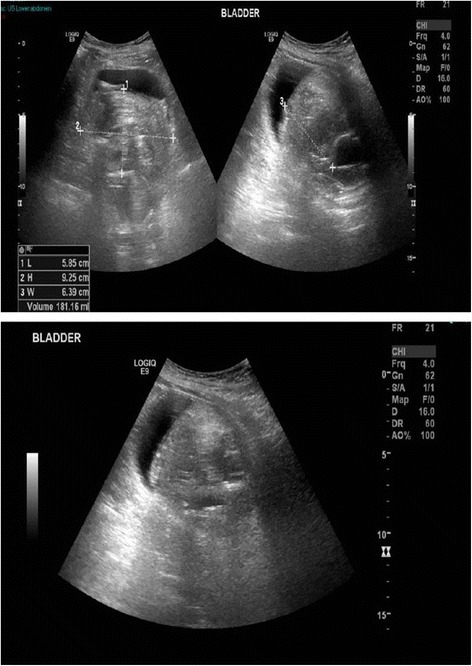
Ultrasound scan of urinary bladder, performed seven days after vesical lithotripsy: Top panel: a large bladder clot measuring 6 ×
